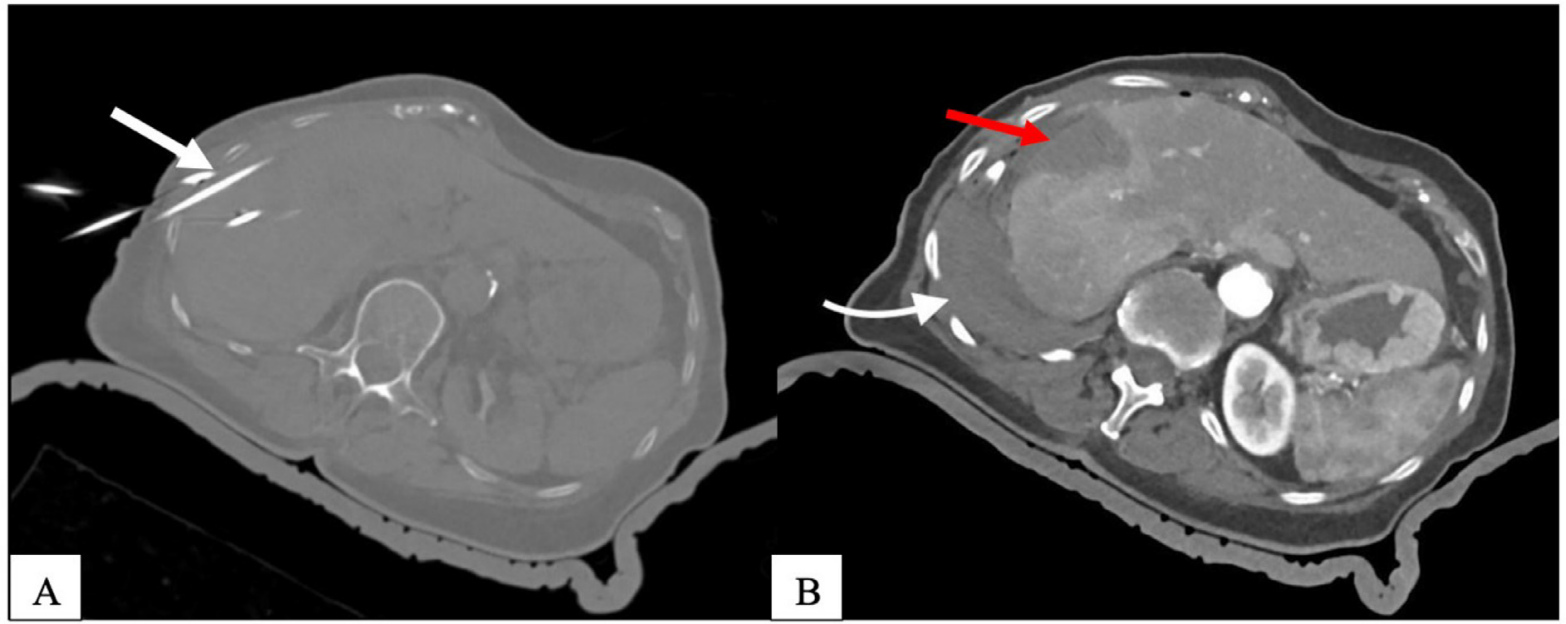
(A-B): CT scan during the coaxiales needles control and after ablation. (Figure 4A axial view) CT scan during the coaxiales needles control and after ablation (Figure 4B axial view) with corresponding necrosis zones in liver segment VIII. Notice the hemothorax (Figure 4B) on the right basal side of the Lung (white arrow).
